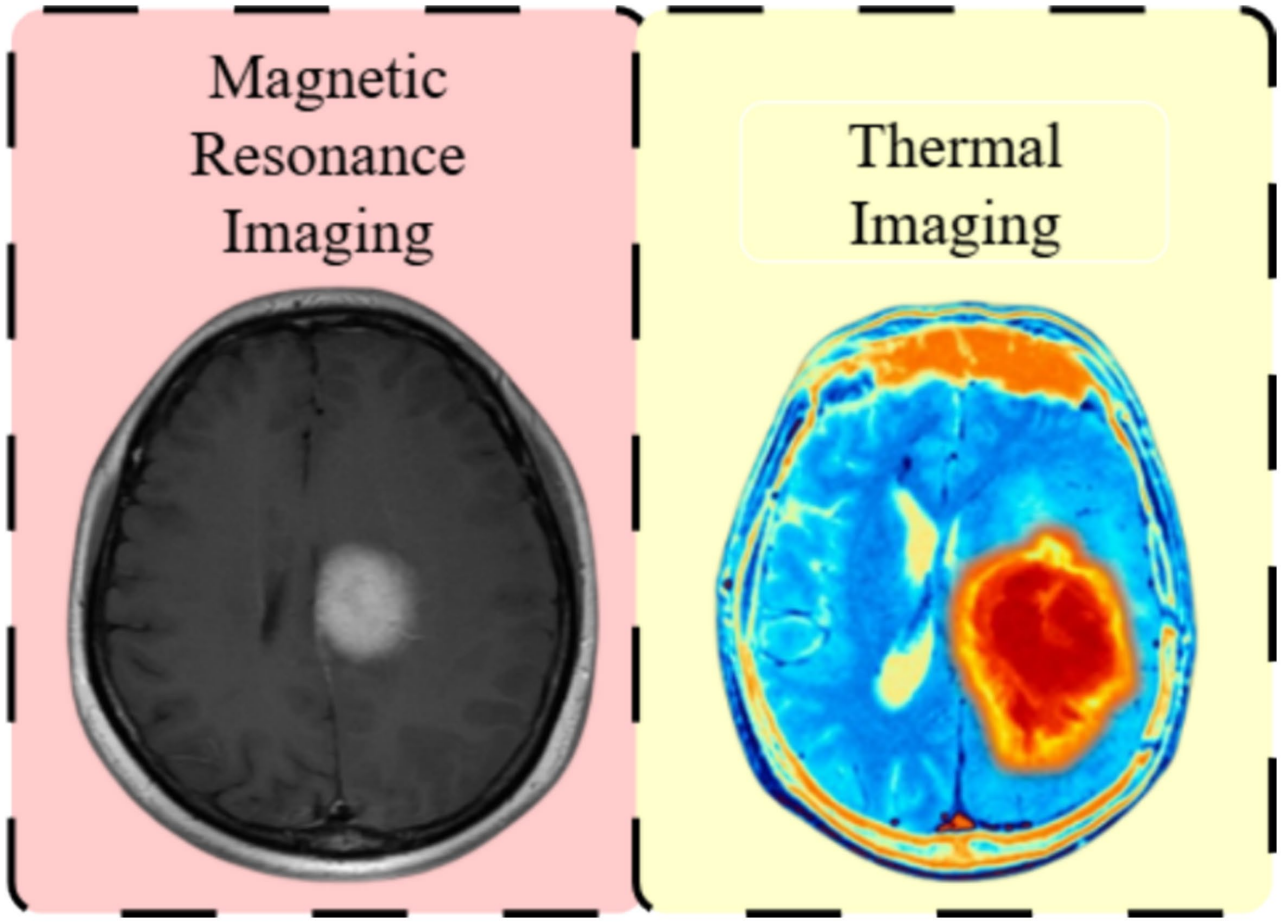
Structural MRI versus thermal imaging for brain tumor analysis. MRI depicts anatomical features, whereas thermal imaging reveals physiological abnormalities associated with tumor activity (Appiah et al., 2024).
